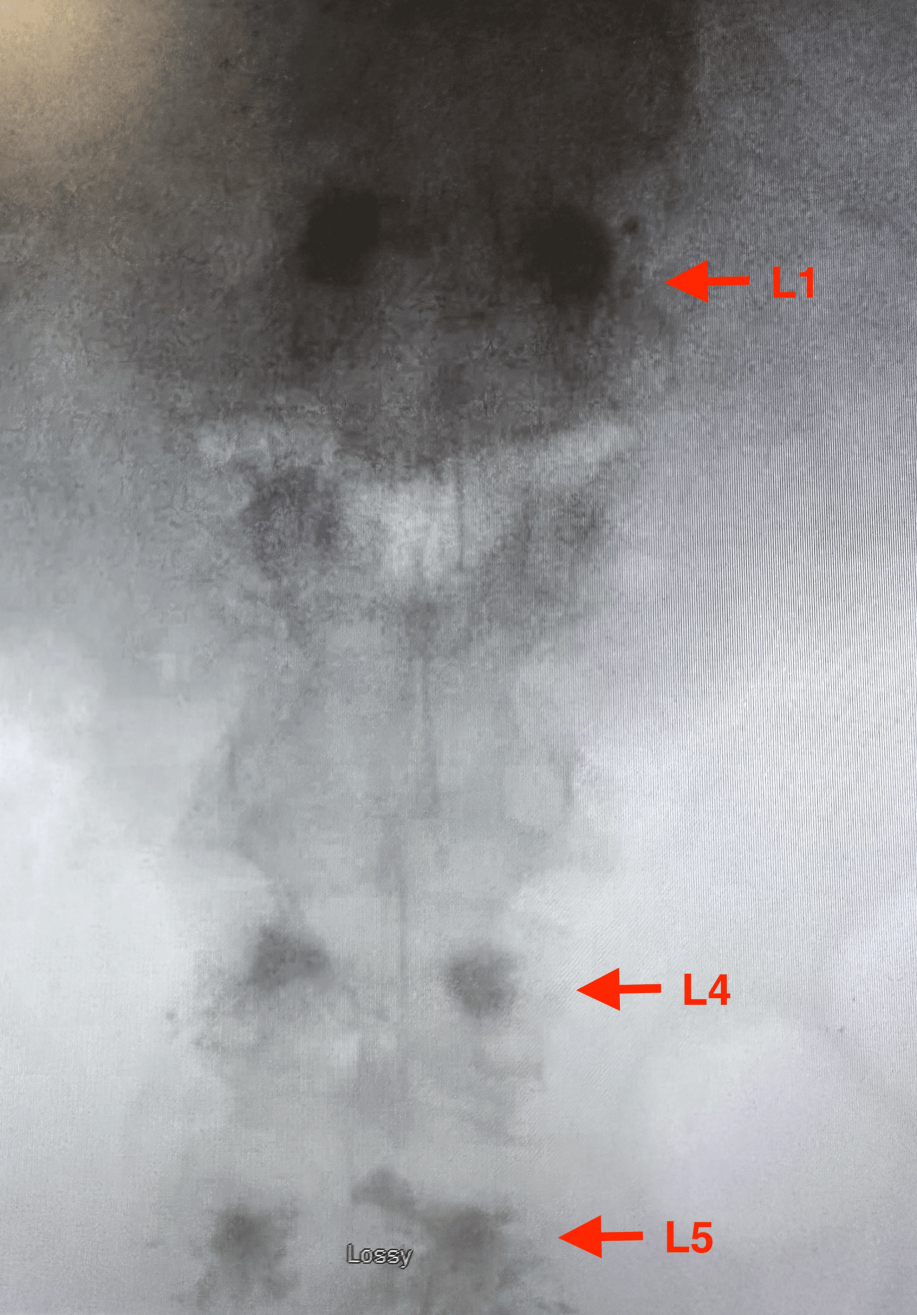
Postoperative anteroposterior lumbar spine radiograph demonstrating radiopaque kyphoplasty cement within treated vertebral bodies L1, L4, and L5 (red arrow). The image appears enlarged due to portable bedside acquisition. Image captured using a smartphone camera due to limitations in accessing downloadable imaging files. All identifiable information was removed.
